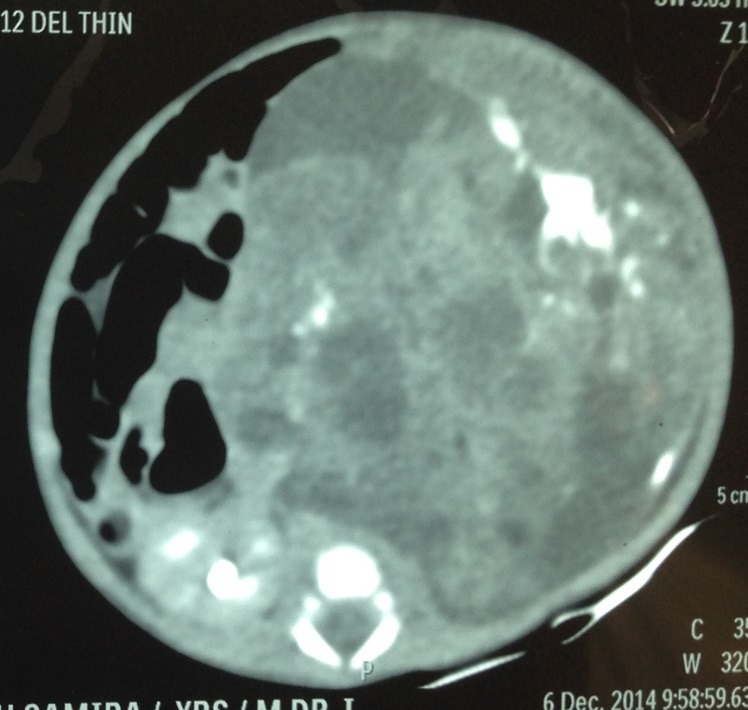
Figure 1: CT scan of the abdomen showing solid cystic tumor in retroperitoneum with foci of calcification
